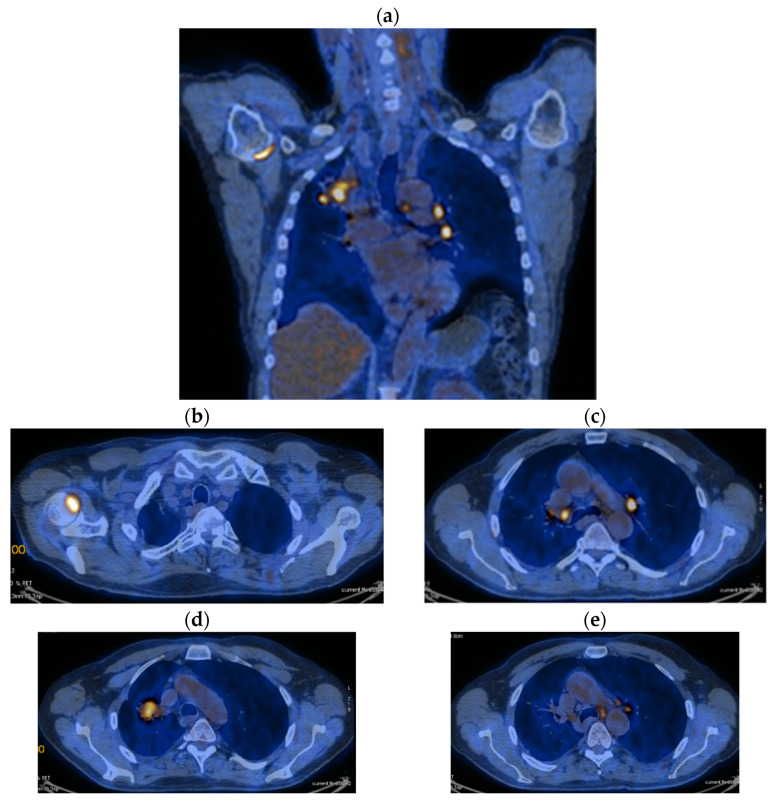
(a–e) PET CT reevaluation.
